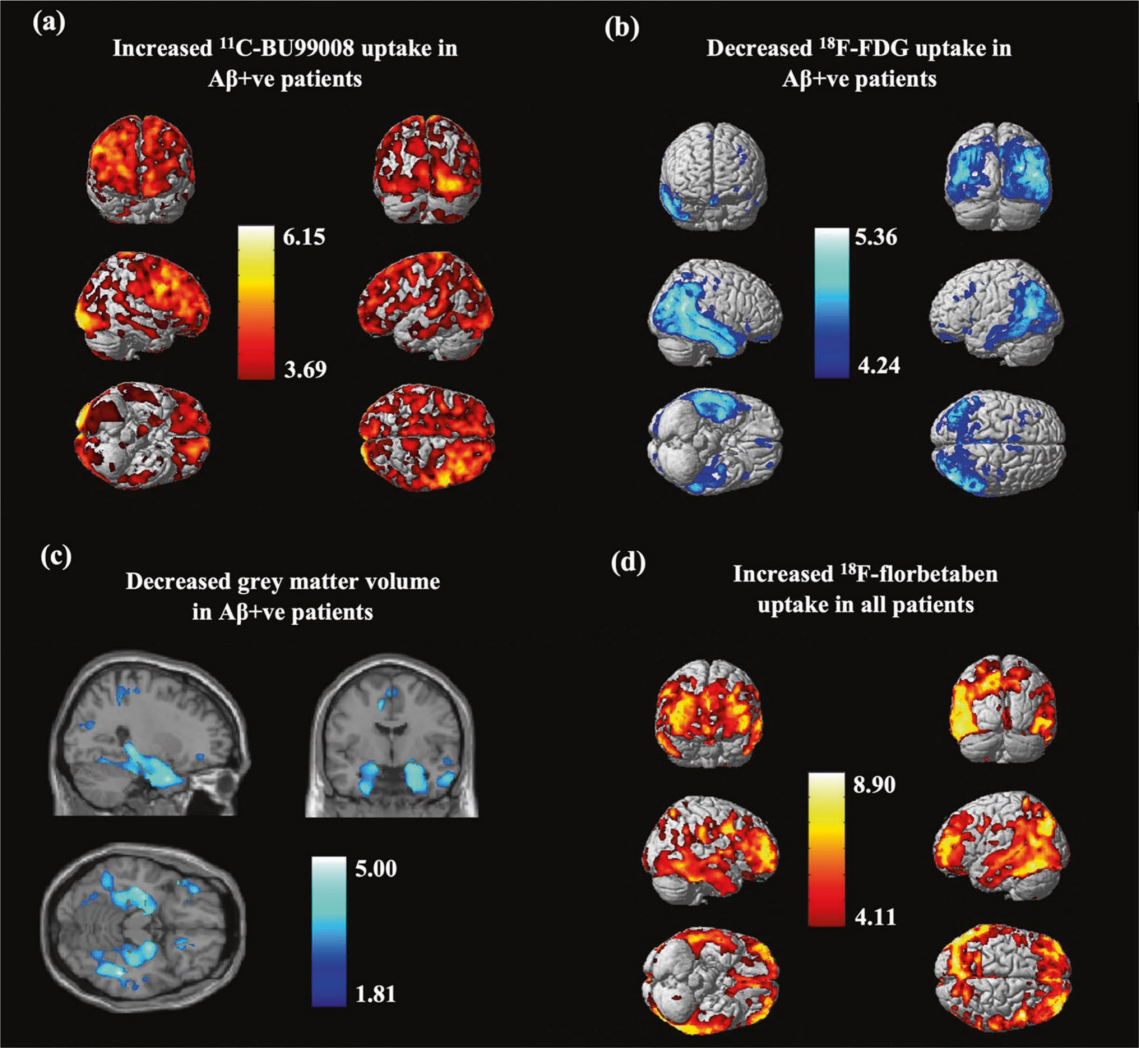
Relationship between [^11^C]BU99008, [^18^F]FDG, gray matter volume and [^18^F]florbetaben in AD patients. This research was originally published in *Mol Psychiatry*. Livingston NR, Calsolaro V, Hinz R, Nowell J, Raza S, Gentleman S, et al. Relationship between astrocyte reactivity, using novel ^11^C-BU99008 PET, and glucose metabolism, grey matter volume and amyloid load in cognitively impaired individuals. Mol Psychiatry. 2022;27:2019-29. http://creativecommons.org/licenses/by/4.0/
